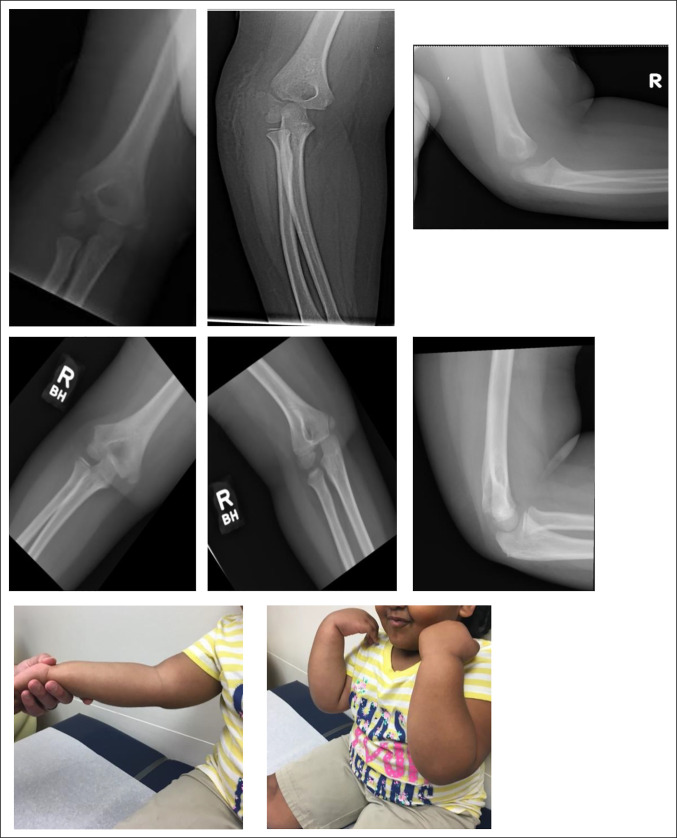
Five-year-old girl: A–C, AP, oblique, and lateral radiographs of initial injury. D–F, Nine months post-op. G and H, Full range of motion after CRPP.
